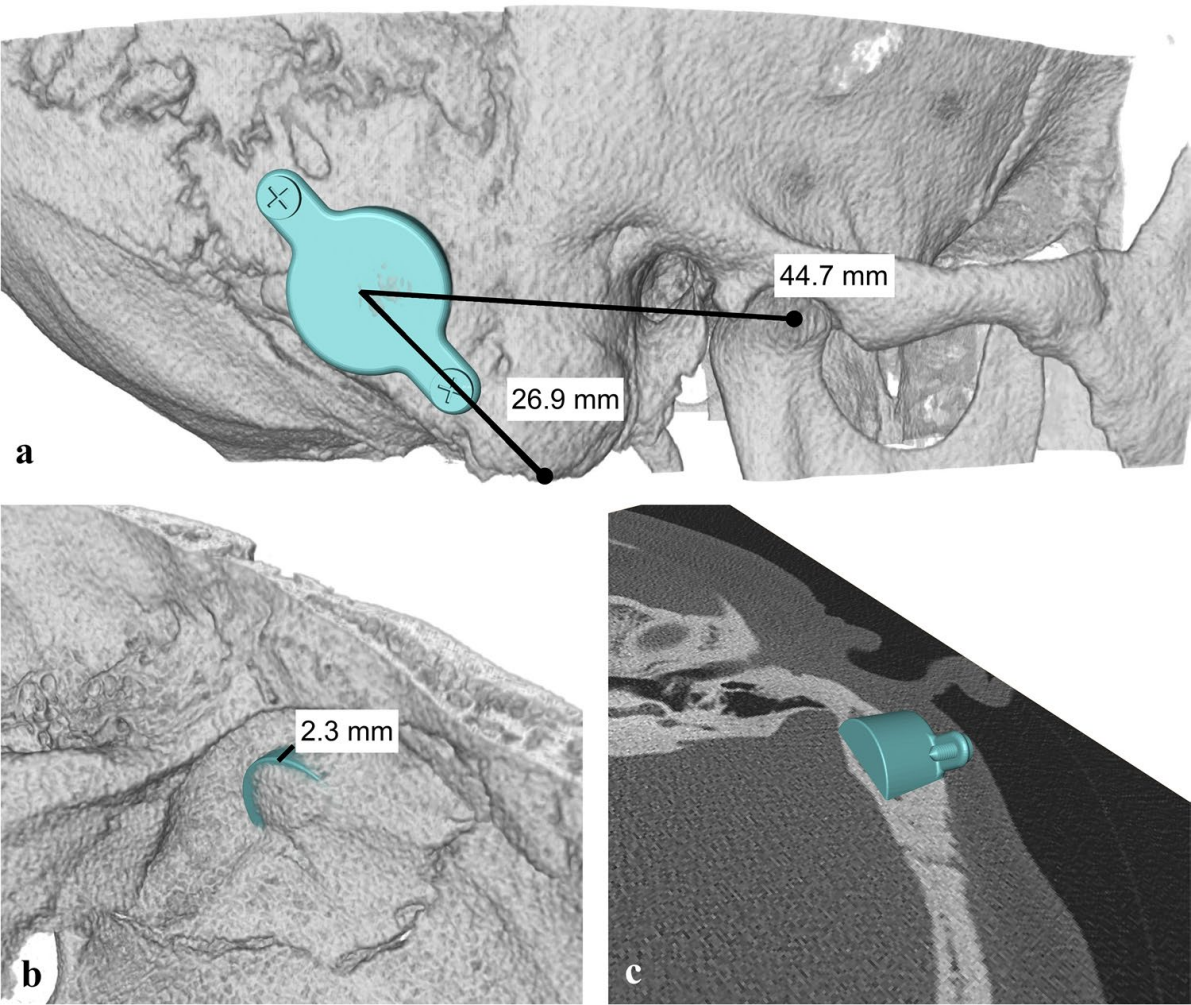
Example of optimal FMT position determined by preoperative 3D planning (“virtual surgery”). a Extracranial view with distances to landmarks for intraoperative transfer. b Intracranial view, where a partial impression of the sigmoid sinus indicates the necessity of the use of lifts. c Verification of the implant position overlying the axial CT scan layer
